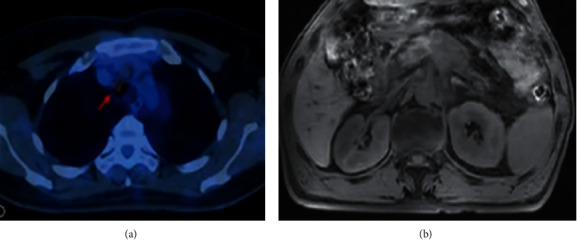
The PET-CT images of two patients. (a) PET-CT image of the pneumonia with pleural effusion patient; (b) PET-CT image of the lung cancer with pleural effusion patient.
